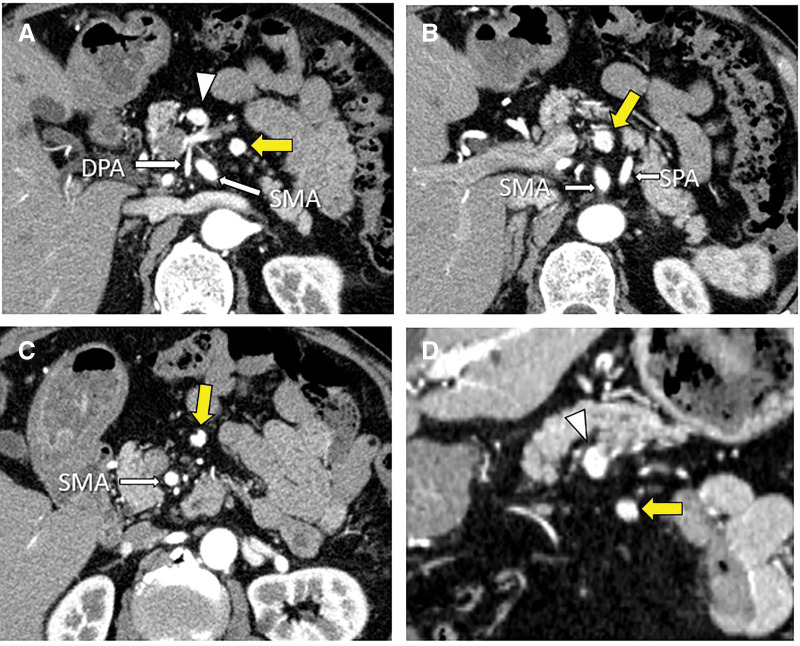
Dynamic contrast-enhanced CT demonstrating a 10-mm hyperenhancing lesion in the pancreatic body (white arrowhead) (A), and 3 similarly enhancing nodules in the transverse mesocolon (yellow arrows) (B and C). (D) Coronal section showing the tumor (arrowhead) and a lymph node (arrow). DPA, dorsal pancreatic artery; SMA, superior mesenteric artery; SPA, splenic artery
